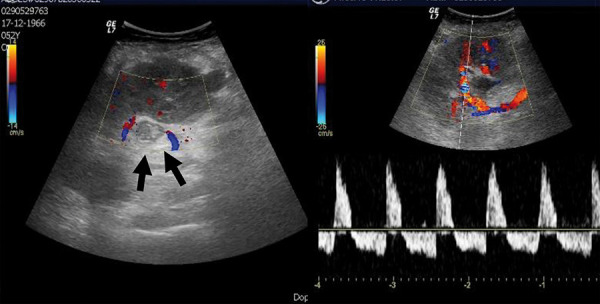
Initial Doppler ultrasonography showing left enlarged renal vein filled with hypoechogenic content, without Doppler signal (black arrow), and onright arterial reversed diastolic flow.
